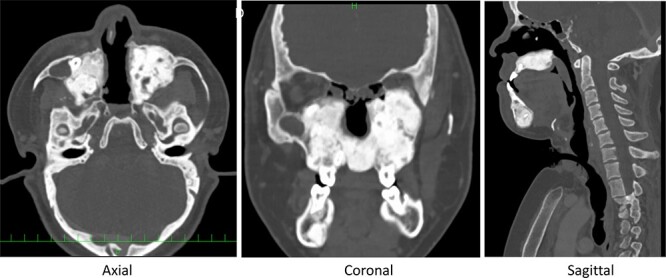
Post-operative CT midline bone has been drilled out, and space for nasal airflow has been widened.
